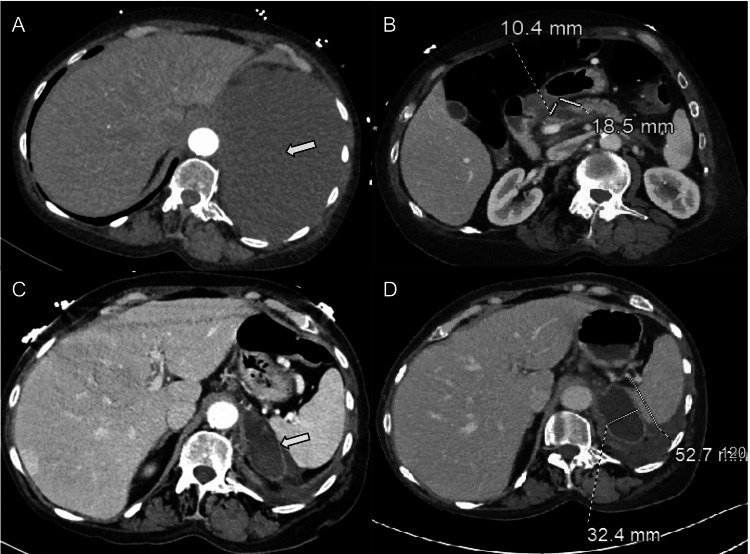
Chronological axial contrast-enhanced CT imaging demonstrating progression of a pancreaticopleural fistula. (A) Initial chest CT demonstrating a large left-sided pleural effusion (arrow) at first admission.
